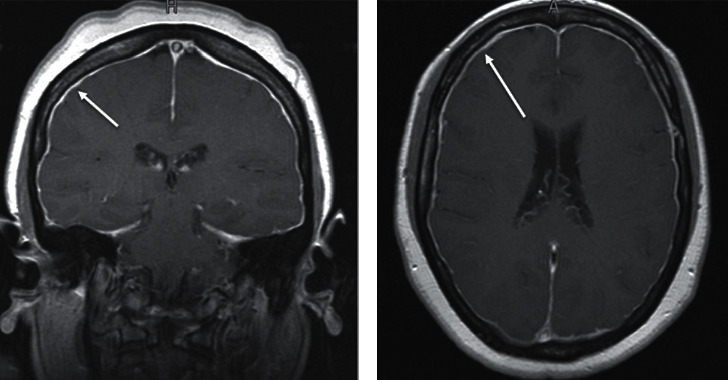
Coronal (a) and axial (b) T1W MRI images with gadolinium enhancement, demonstrating diffuse pachymeningeal enhancement (arrows).
